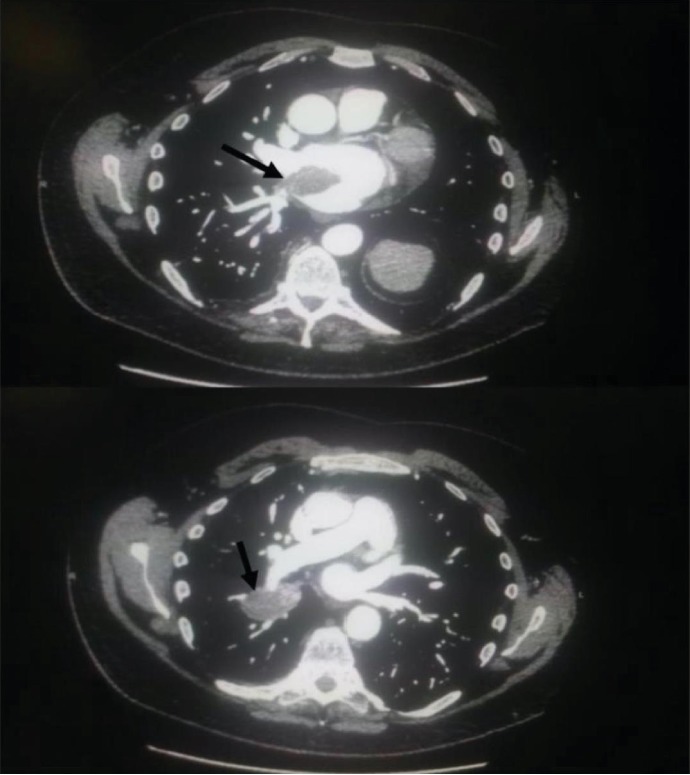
Computed tomography imaging showing a tumor in the lung parenchyma with invasion of the left atrium (black arrows).
